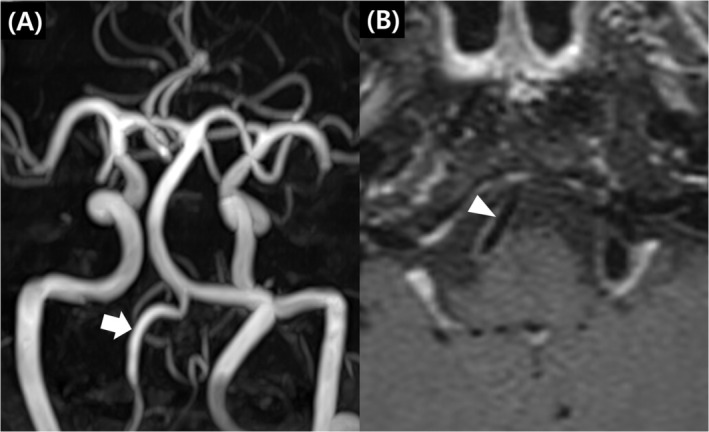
Posttreatment imaging at the 11‐month follow‐up (December 2, 2025). (A) Frontal MIP MRA showing morphological restoration of the right V4 vertebral artery with normal flow, symmetric caliber, and no residual stenosis. (B) Axial T1‐weighted high‐resolution vessel wall MRI showing resorption of the intramural hematoma and normalization of the arterial wall.
